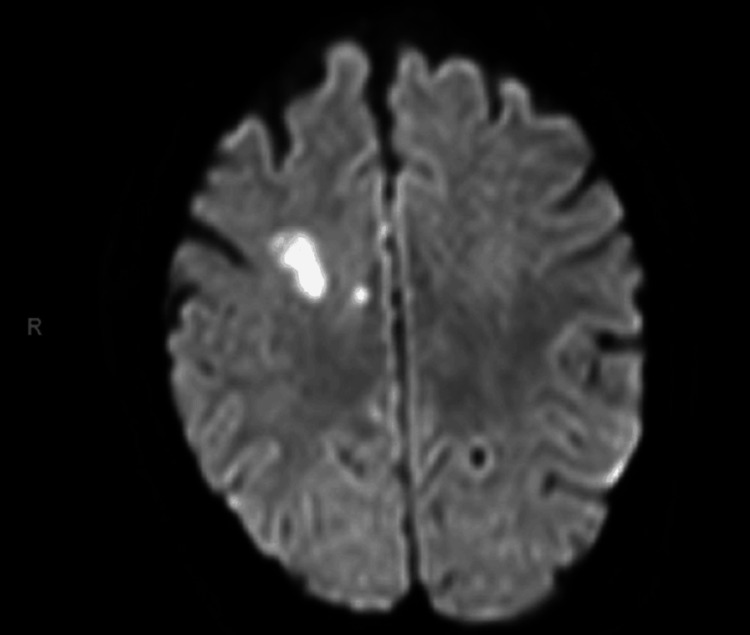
Initial MRI brain showing acute infarcts in the right high frontoparietal lobe and few other tiny infarcts of varying ages with restriction diffusion on DWI/ADC scans DWI/ADC: Diffusion-weighted imaging/apparent diffusion coefficient
